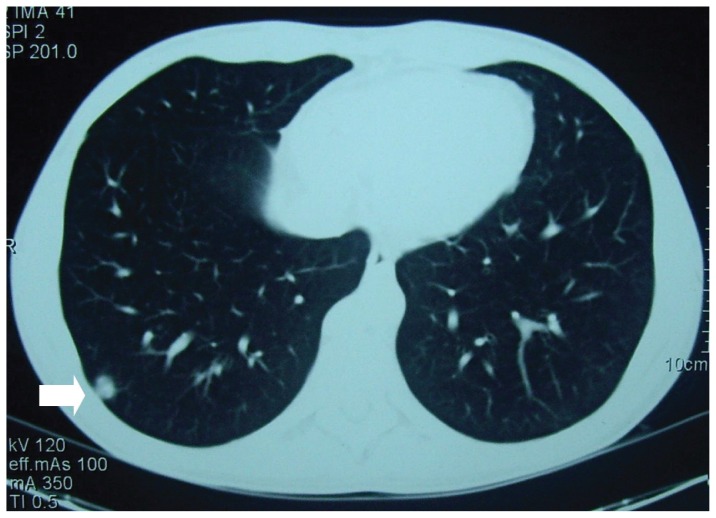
CT-scan of the chest showing a well-circumscribed mass in the right lung basis (arrow).
